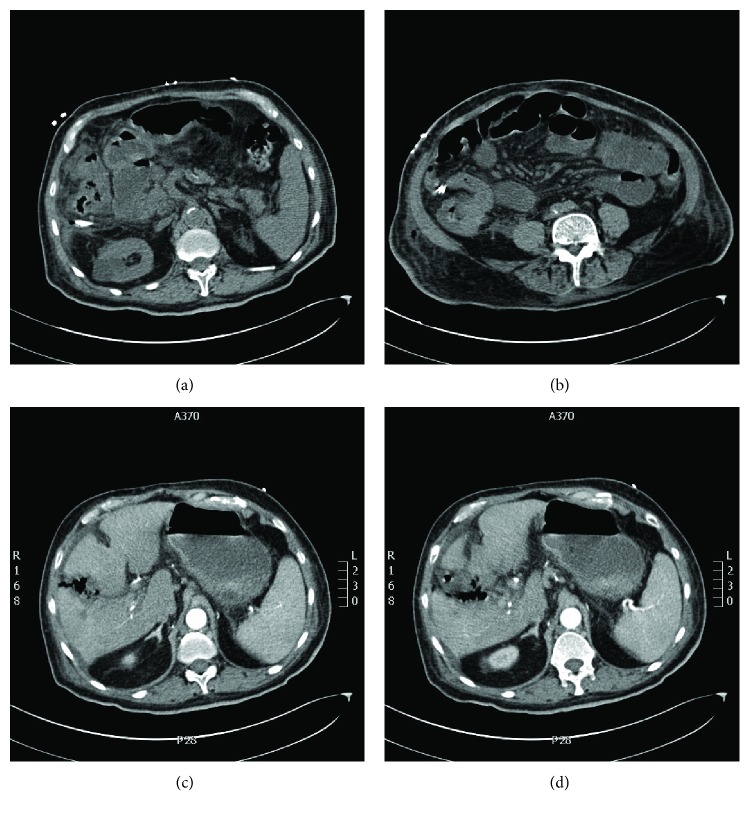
(a, b) CT Abdomen showing cecal mass. (c, d) CT abdomen showing post cholecystectomy changes.
